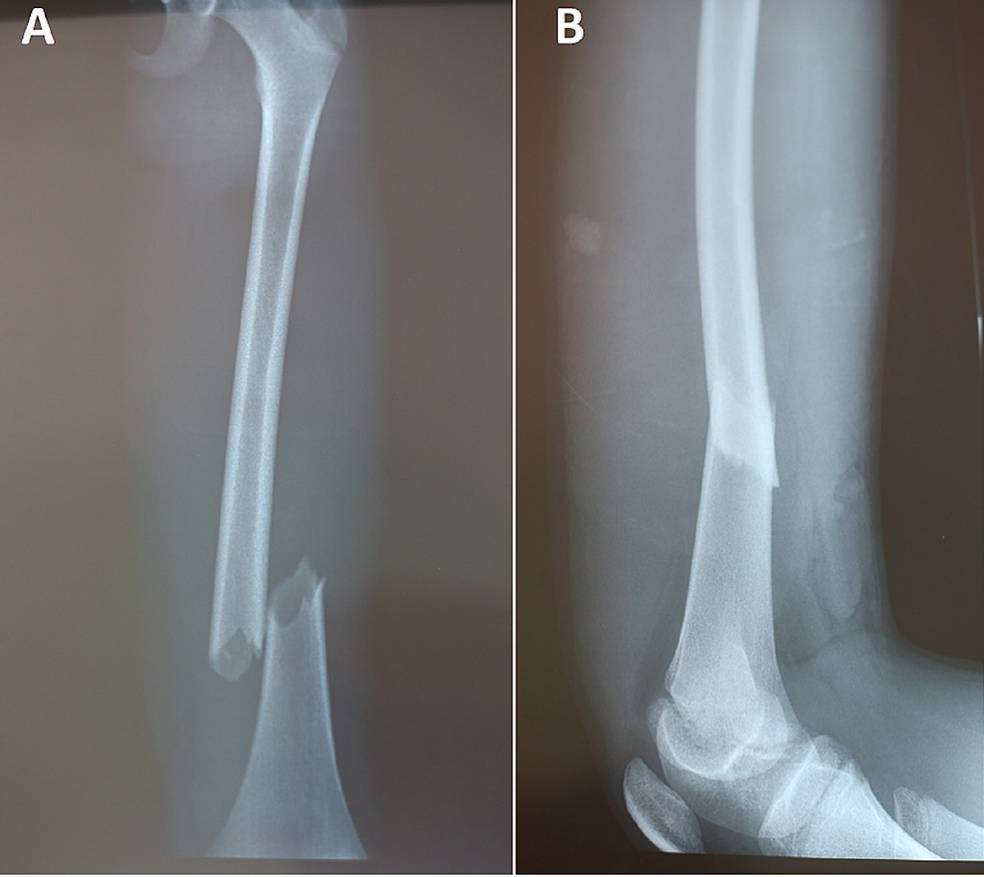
Right thigh x-ray imaging showing an overlapping and displaced distal diaphyseal fracture of the femur on anteroposterior (A) and lateral views (B)
